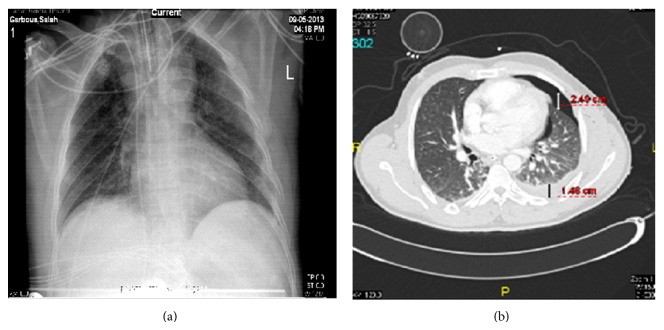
(a) Chest radiograph and (b) corresponding CT scan of patient with 24 mm pneumothorax and 14 mm hemothorax on left side not identified on chest X-ray.
